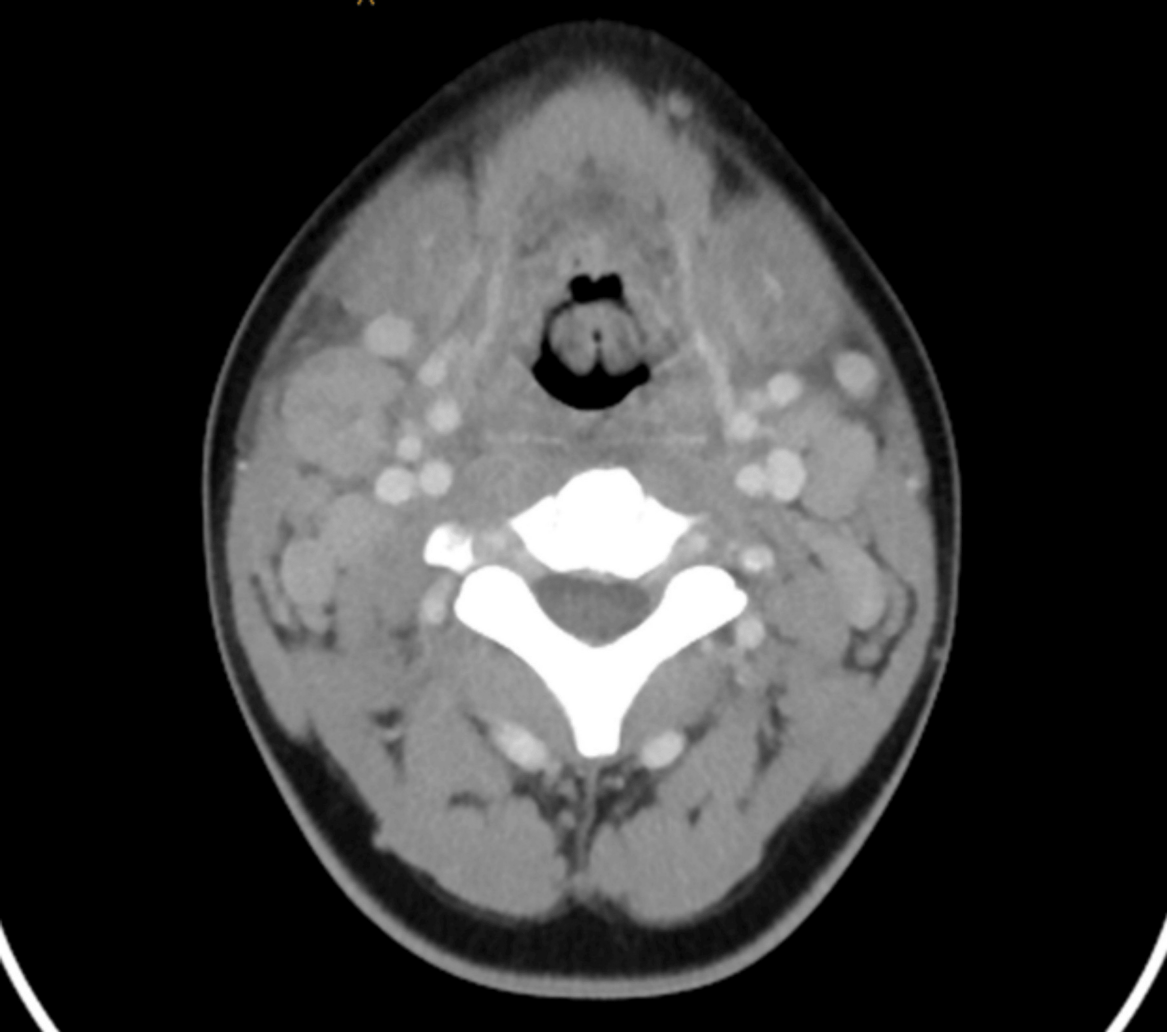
Initial CT obtained on day one showing epiglottis
